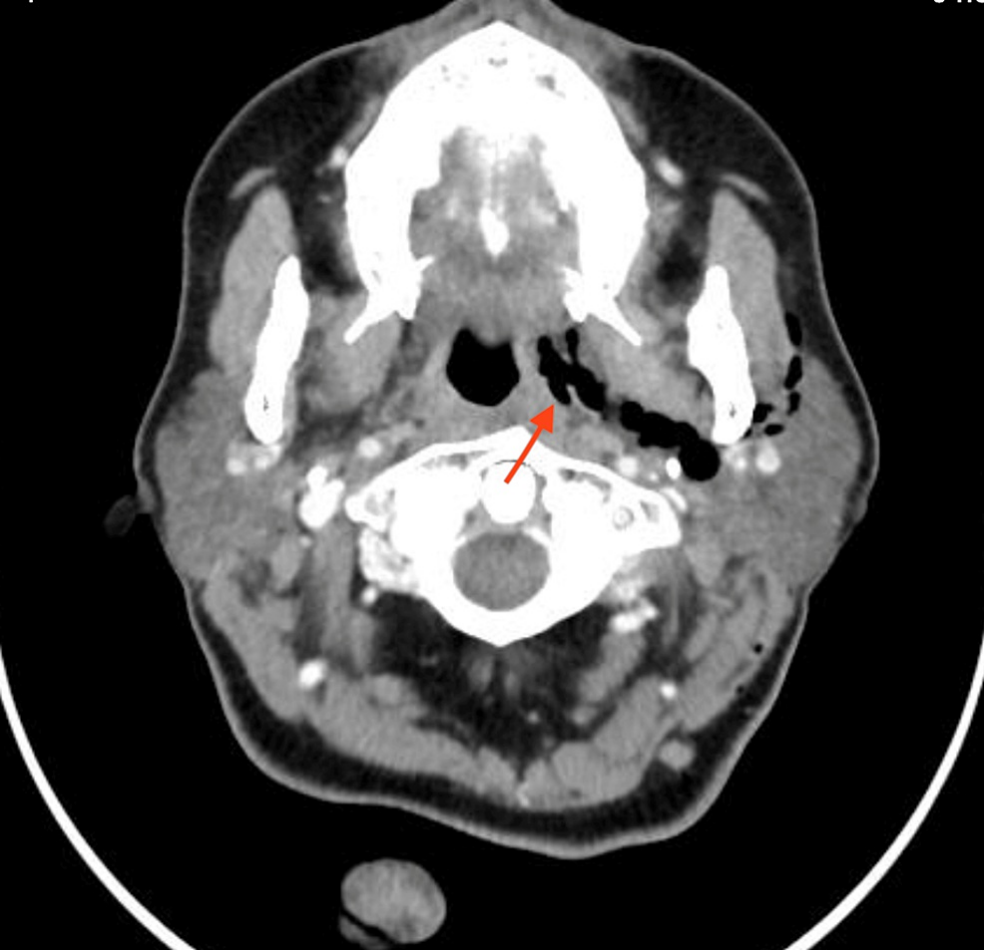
Computed tomography (CT) with contrast, axial view The arrow pointing to the area of extension of air to the left parapharyngeal space.
